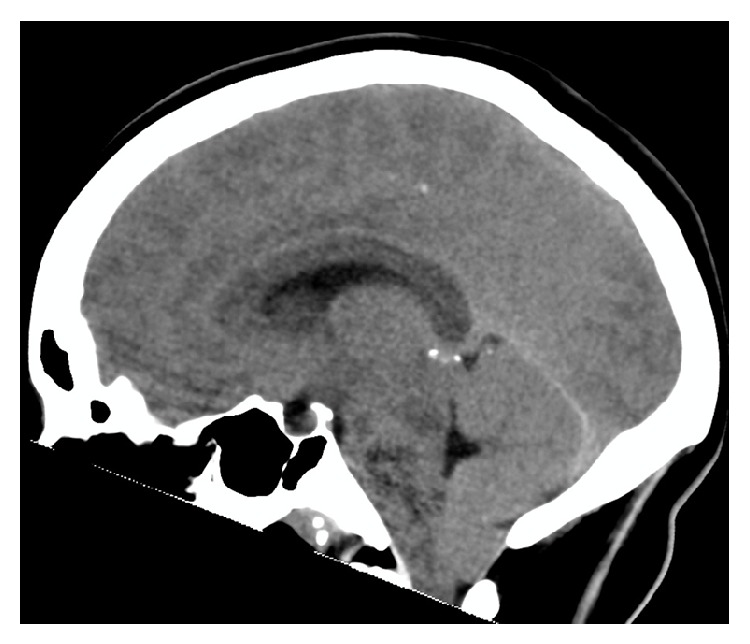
Sagittal CT scan demonstrating 10 mm of tonsillar descent with posterior fossa crowding.
